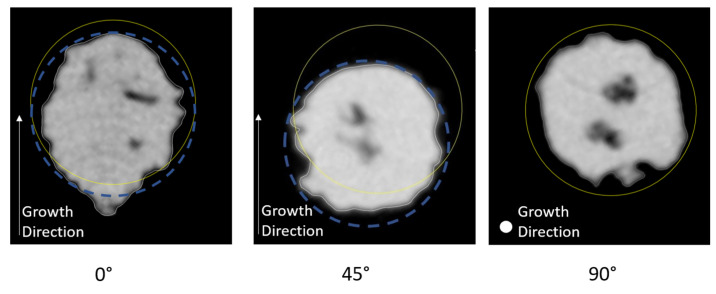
X-ray images of cross-section variation with orientation and comparison with the nominal cross-section.
